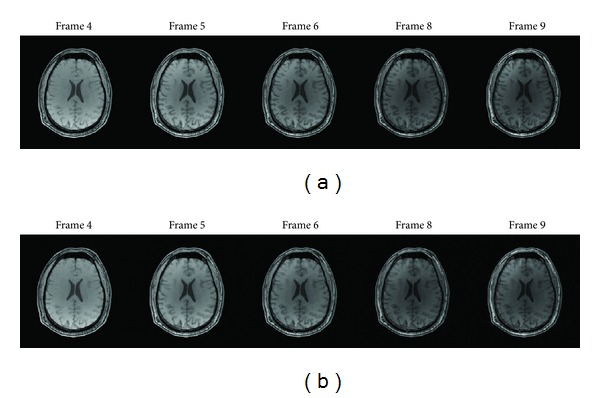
The reconstruction results of an MRI sequence with 20% of the fully sampled data. (a) Target images to be reconstructed. (b) Corresponding reconstructions by the proposed method.
